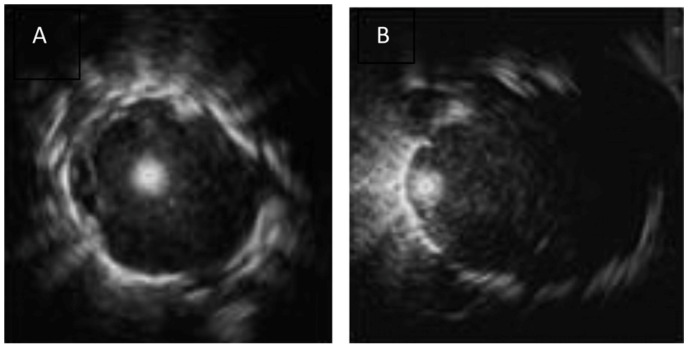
Concentric radial endobronchial ultrasound (EBUS) image of a lesion with the probe centered and surrounded by the lesion (A). Eccentric radial EBUS image of a lesion with the probe positioned at the edge of the lesion (B).
